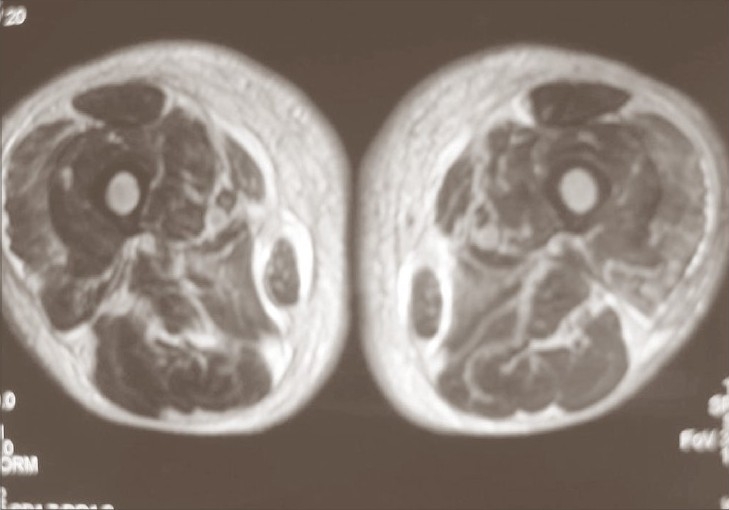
T2-weighted MRI with fat suppression technique shows thickening of the skin and edema with hyperintense signal that involves the muscles of both thighs, predominantly the left one
